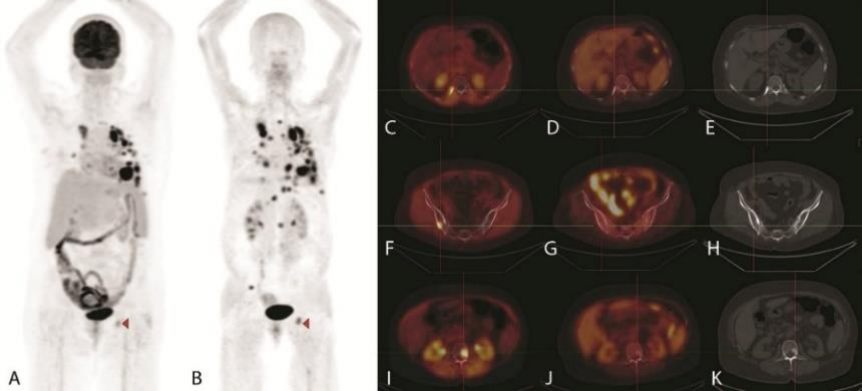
A 59-year-old female, known case of breast cancer, status post lumpectomy and chemoradiotherapy. [^18^F]FDG and [^68^Ga]Ga-FAPI-46 MIP images (A and B, respectively) demonstrate multiple lung and mediastinal lymph node metastases. The noteworthy point was the presence of only one hypermetabolic bone lesion in the left ischium in the [^18^F]FDG images (A, arrow head), while in the [^68^Ga]Ga-FAPI-46 images, more extensive bone metastases are seen, including left ischium (B, arrow head), right 12^th^ rib (C, with corresponding [^18^F]FDG PET/CT fusion and CT images, D and E), right iliac bone (F, with corresponding [^18^F]FDG PET/CT fusion and CT images, G and H), L2 vertebra (I, with corresponding [^18^F]FDG PET/CT fusion and CT images, J and K)
